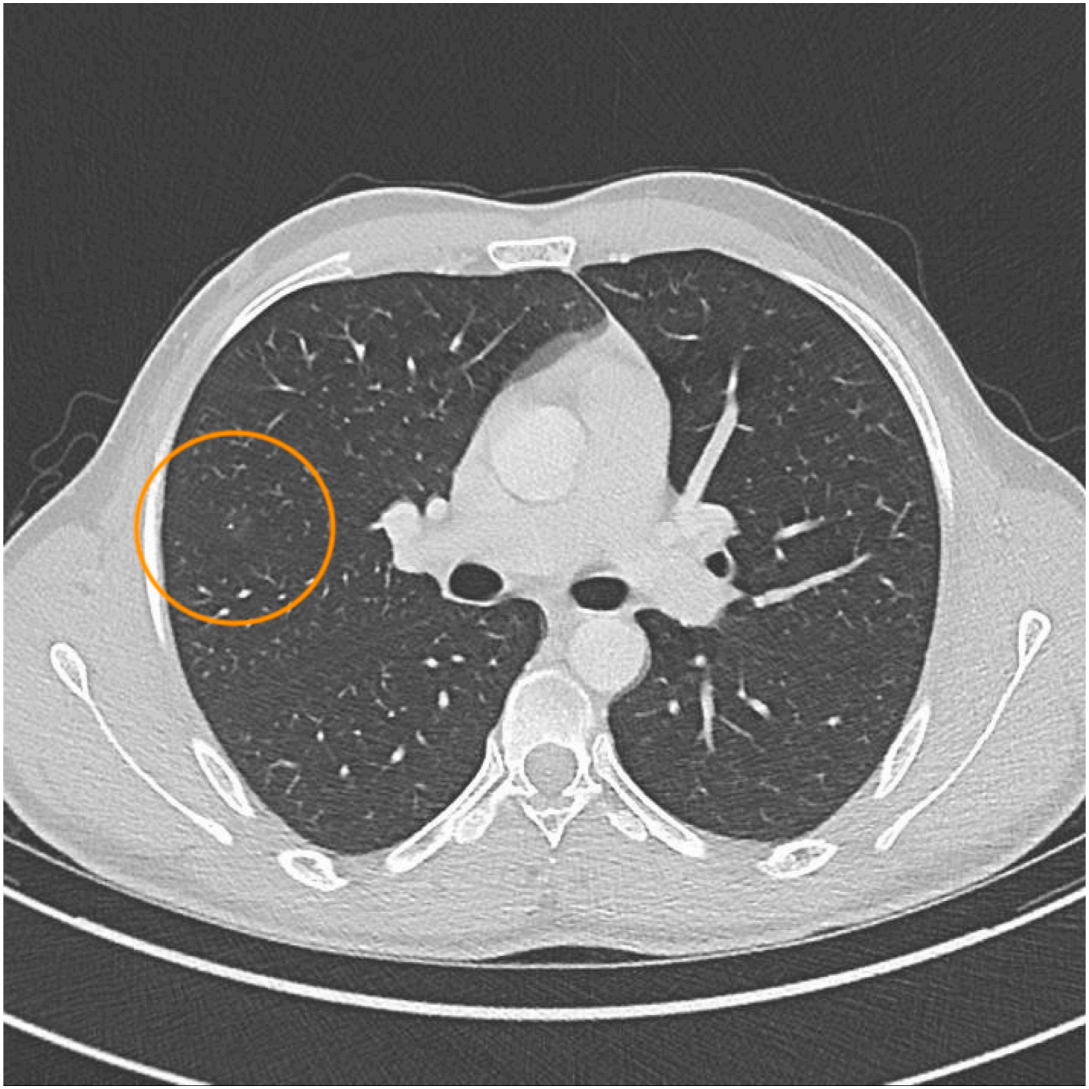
CT thorax at initial presentation showing small granuloma and surrounding ground glass opacity in the right middle lobe. CT = computed tomography.
